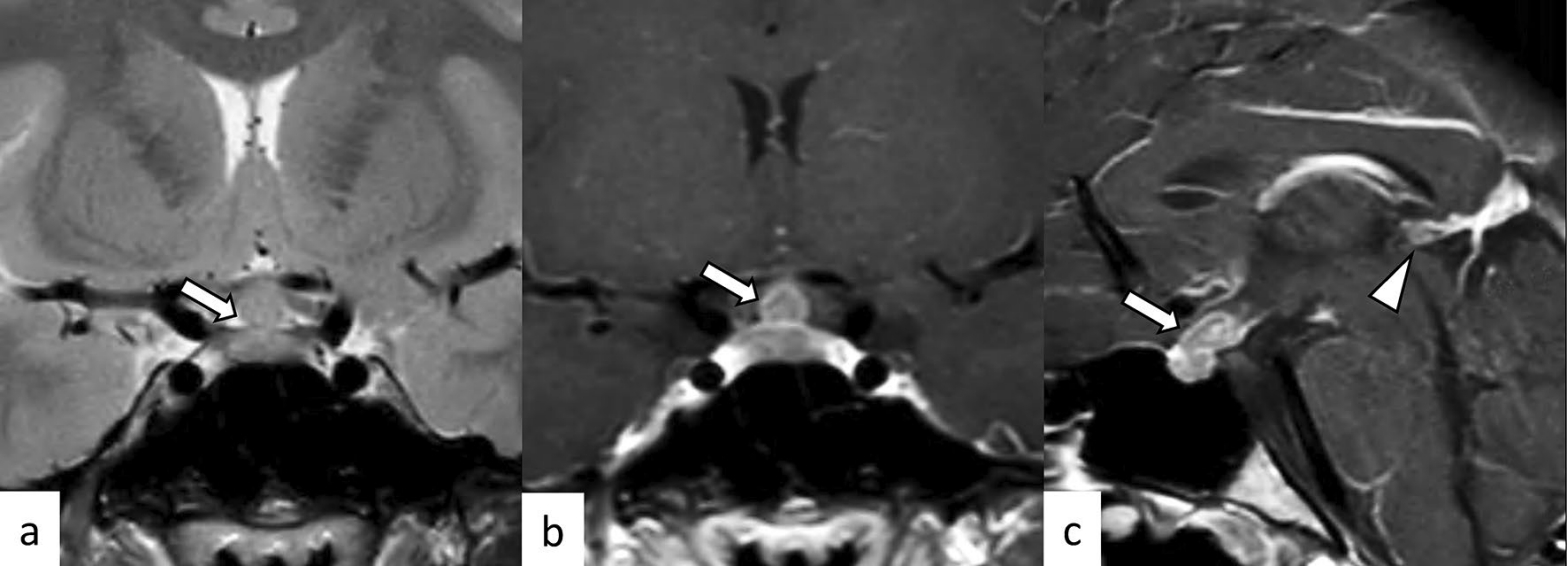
A 17-year-old woman with germinoma involving the pituitary gland and pineal gland. MRI shows enlarged pituitary gland and stalk with T2-weighted hyperintensity (a, arrow) and heterogeneous enhancement (b, c, arrows). A pineal gland lesion is also demonstrated (c, arrowhead)
